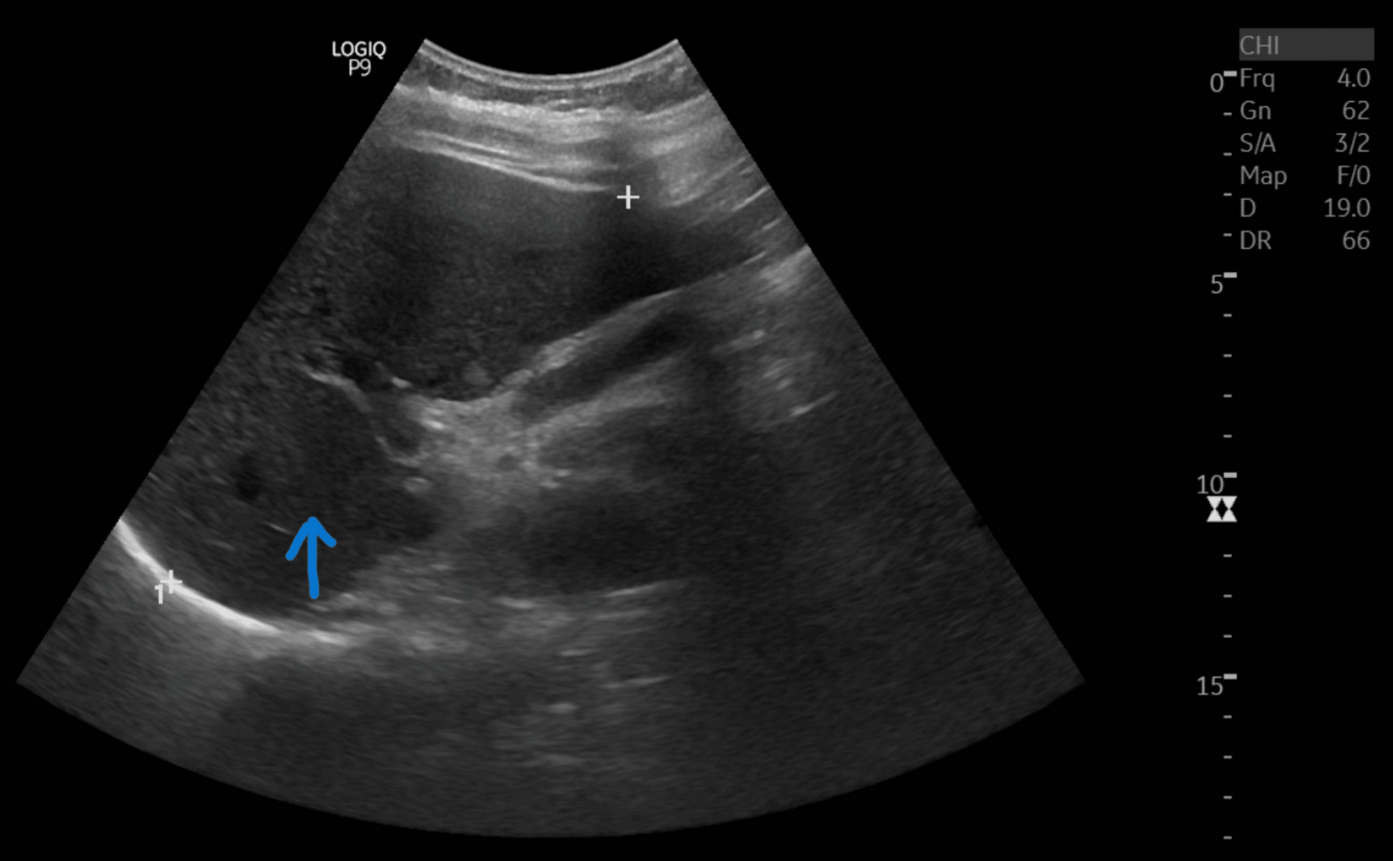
Ultrasound abdomen showing Grade II fatty liver
